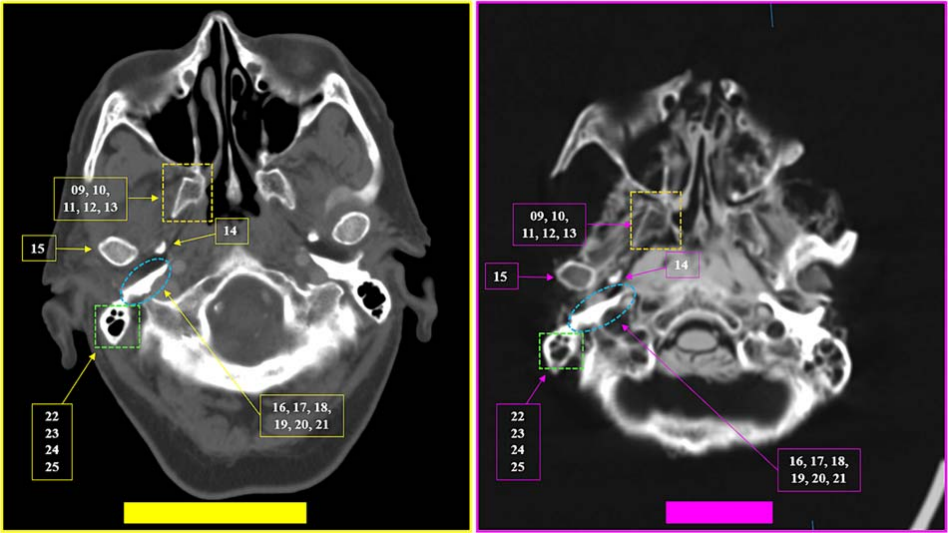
Multiple coinciding points in the cranial anatomical points and in the morphology arrangement of the right mastoid cells (left inferior square) in the antemortem (left) and postmortem (right) CT scans in axial sections.
